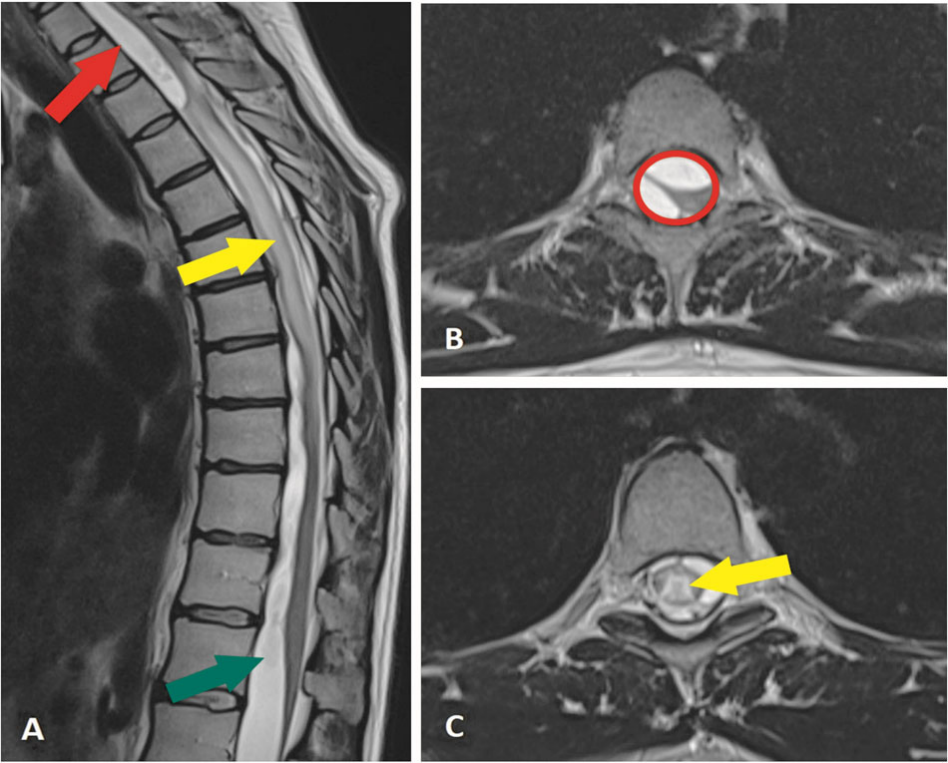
Preoperative T2 sequence MRI images in the (A) sagittal and (B) axial axis of thoracic spine showing dural adhesions (red circle) and septations, more pronounced at T2-T3 level, with arachnoid cysts (red and green arrows) above and below this level; (C) Spinal cord deformity and dislocation, with slightly widened central canal suggesting syringomyelia (yellow arrow) and some vasogenic oedema in spinal cord central parts.
